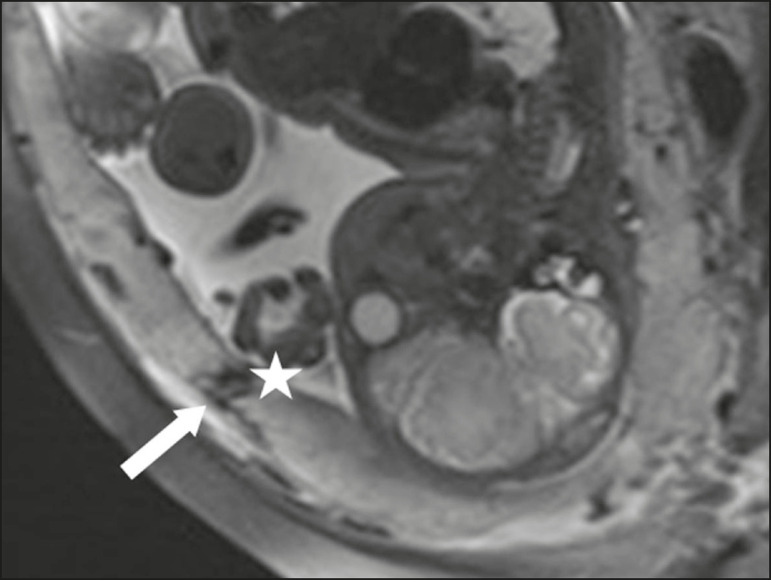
Sagittal T2-weighted MRI sequence showing placental recess and dark bands (star).
